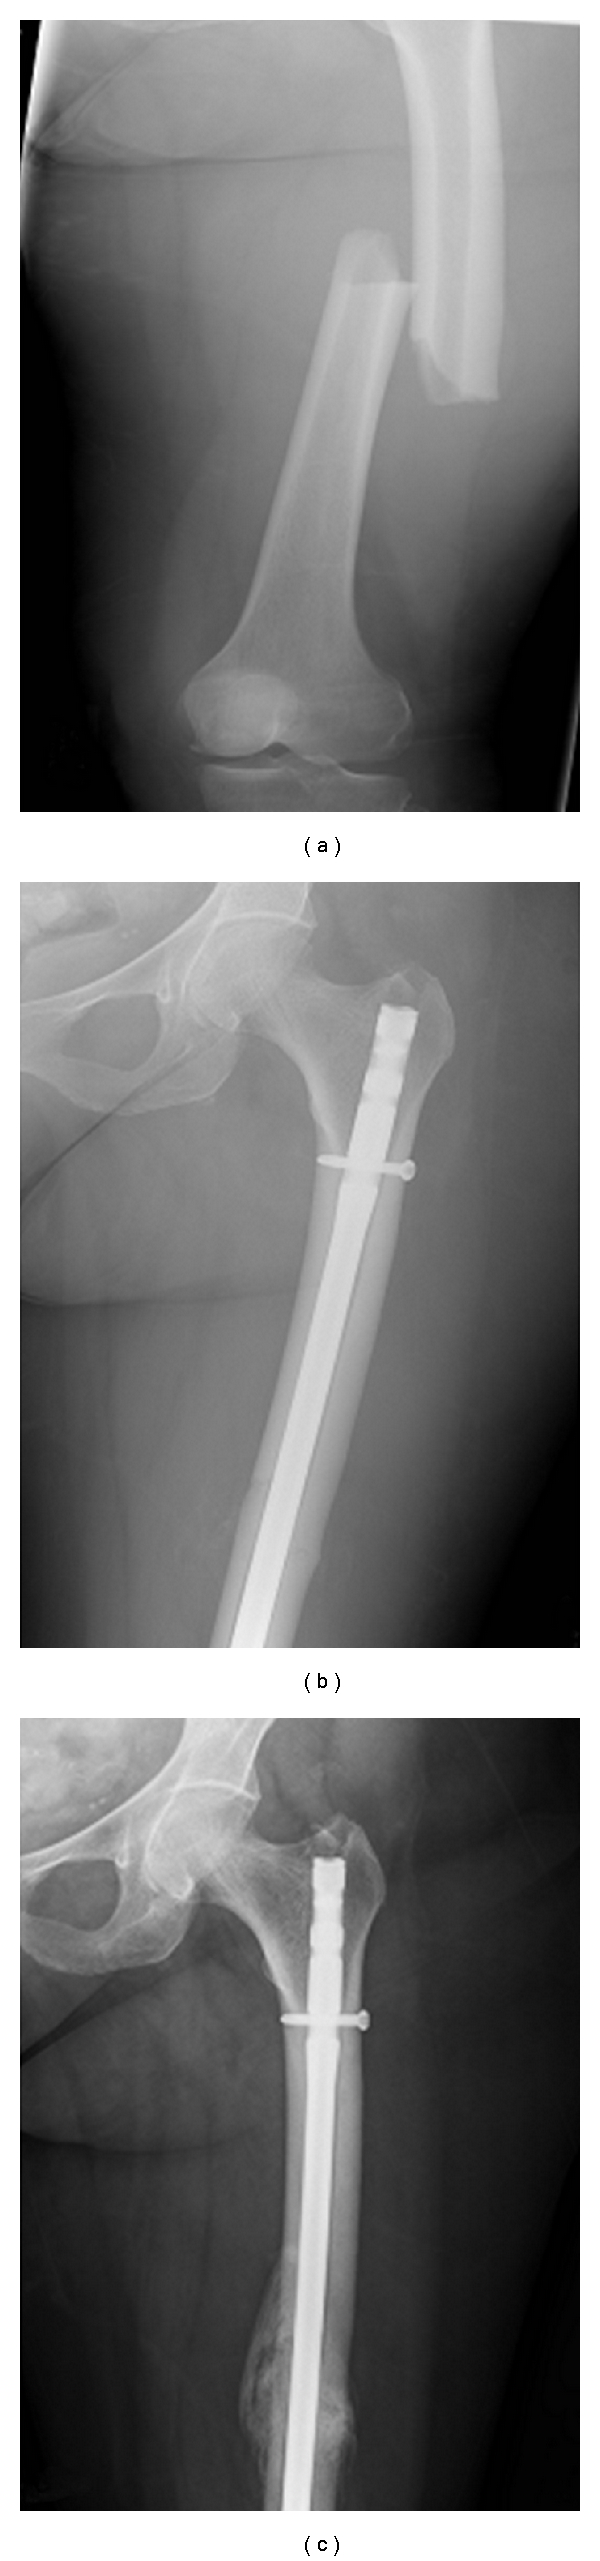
Fracture left femur at the level of stress fracture site, immediate postoperative, and three months postoperative radiographs.
